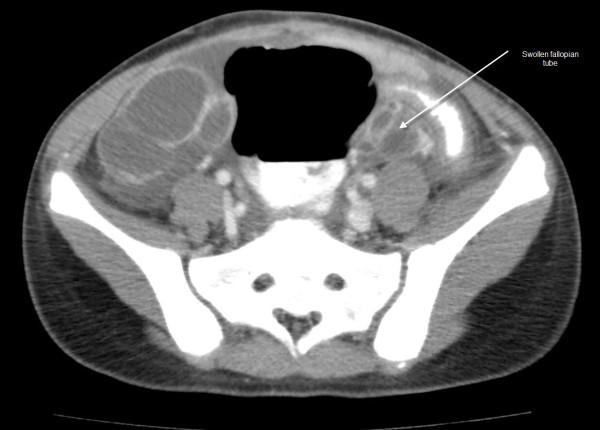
CT scan of the abdomen and pelvis with intravenous and oral contrast. Bilateral dilated fallopian tubes with pronounced wall enhancement are shown.
